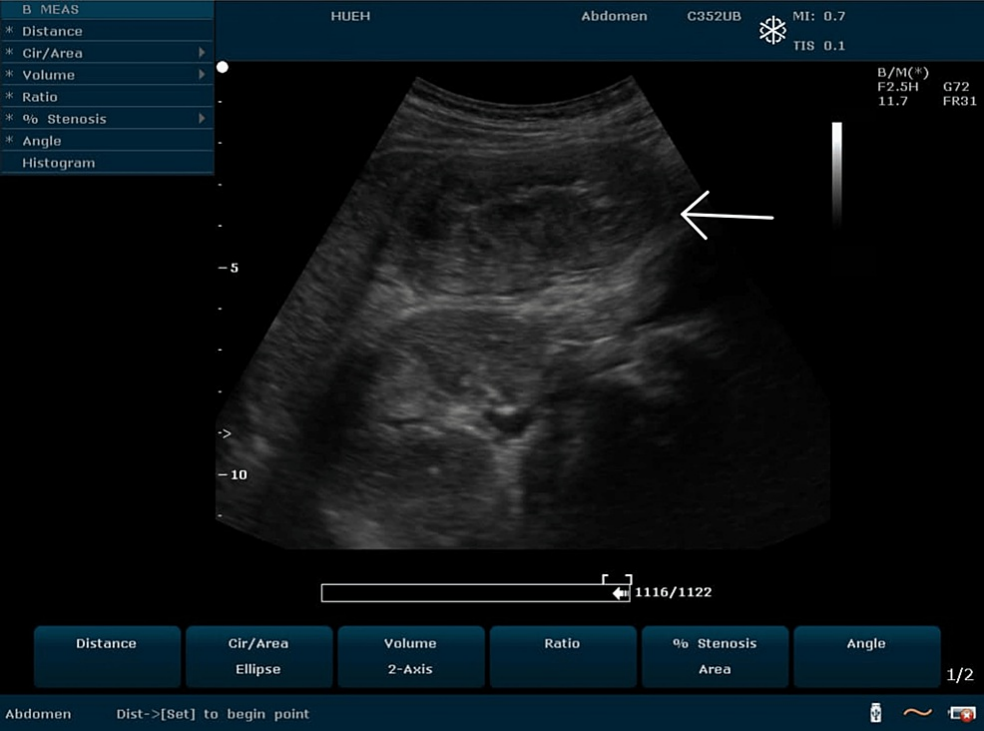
Intestinal mass Long-axis 2-6 MHz ultrasound image obtained over the right lumbar region showing a pseudokidney sign
